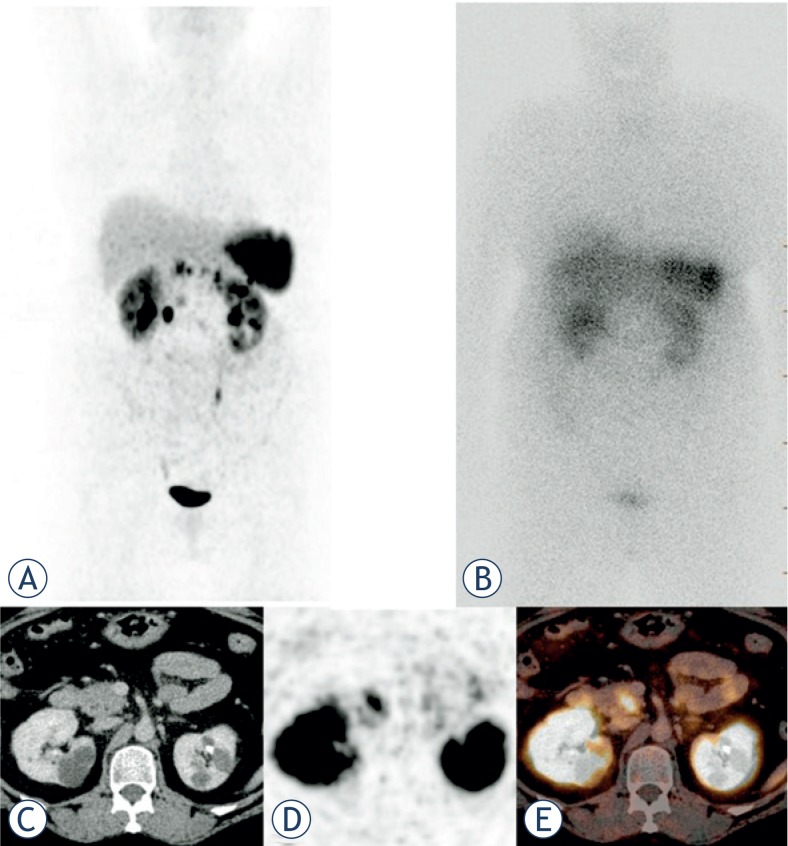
Patient with MEN1 and several NET lesions in the Ga-68 DOTATOC MIP(A), which were not visible in the In-111 octreotide scintigraphy performed a few days before (B), Ga-68 DOTATOC PET/CT images of the lesion in the pancreatic head (C–E).
